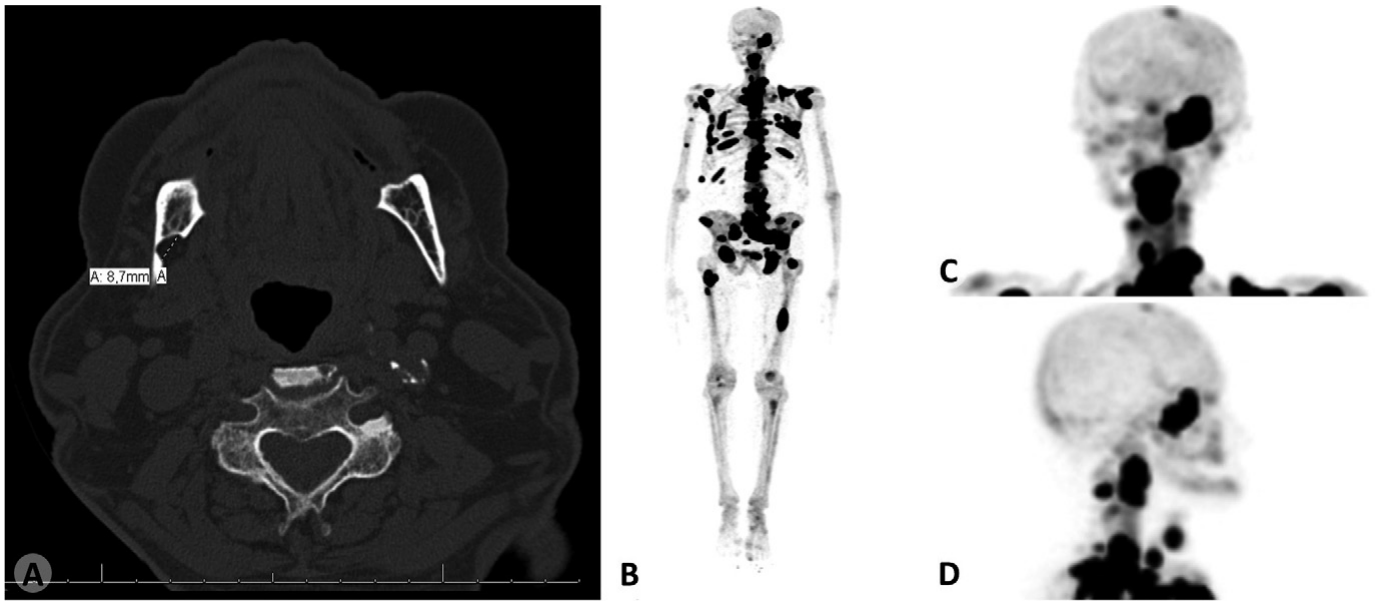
(A) Axial CT view showing the lingual bone depression. (B) Multiple bone metastases evidenced by F-18 radionuclide (dark areas) in a total body bone scintigraphy. (C-D) Detail, showing the lack of hyperconcentration in the correspondent right posterior mandibular area of frontal and lateral cranium views.
